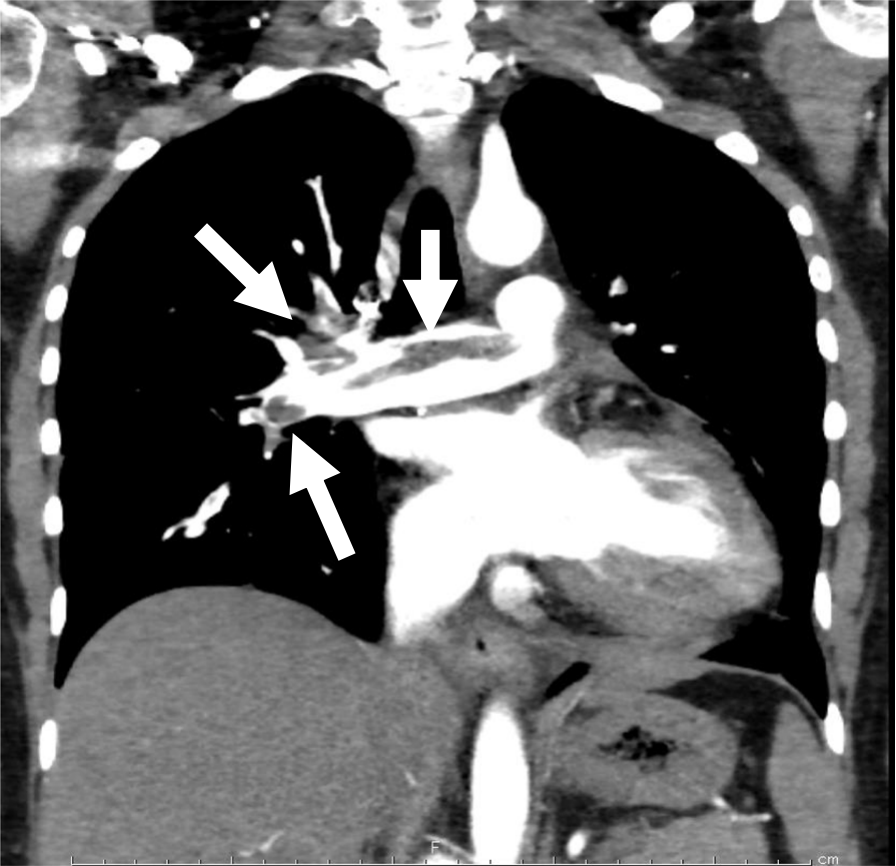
Computed tomography showing a thrombus (arrow) in the pulmonary artery
